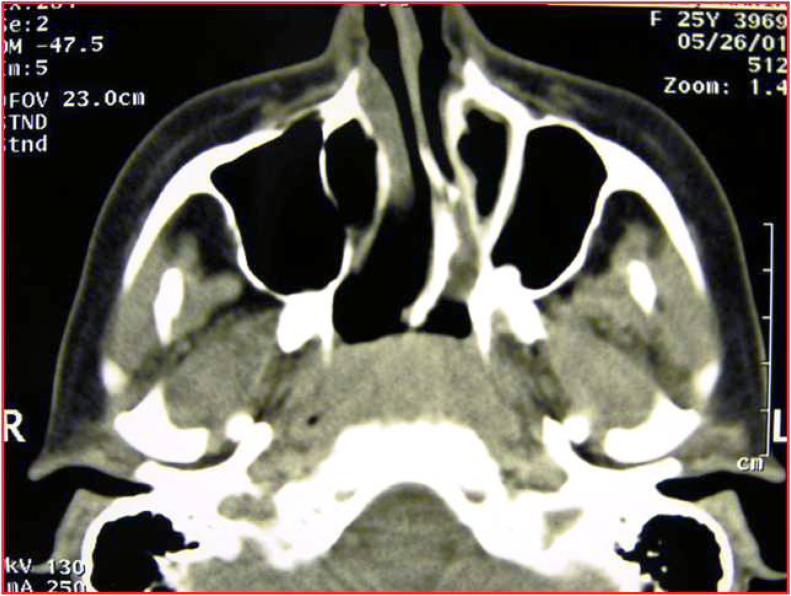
Axial CT scan, monolateral left mixed choanal atresia.
